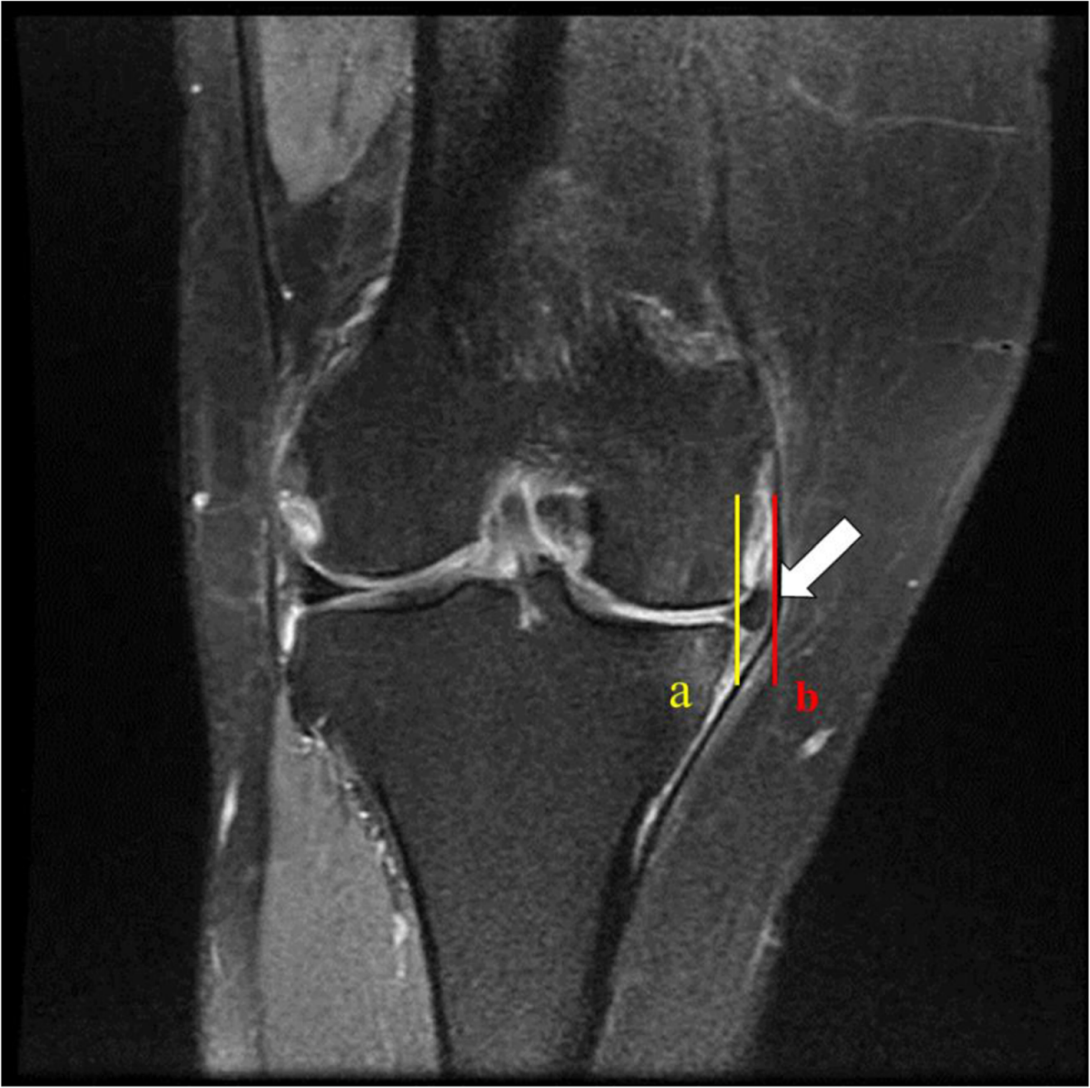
Measure the length between the vertical line (a) and the line (b), which represents the outer edge of the meniscus, in the medial side of the tibial proximal articular surface in the coronal plane magnetic resonance imaging, femoral medial condyle mid cut image
